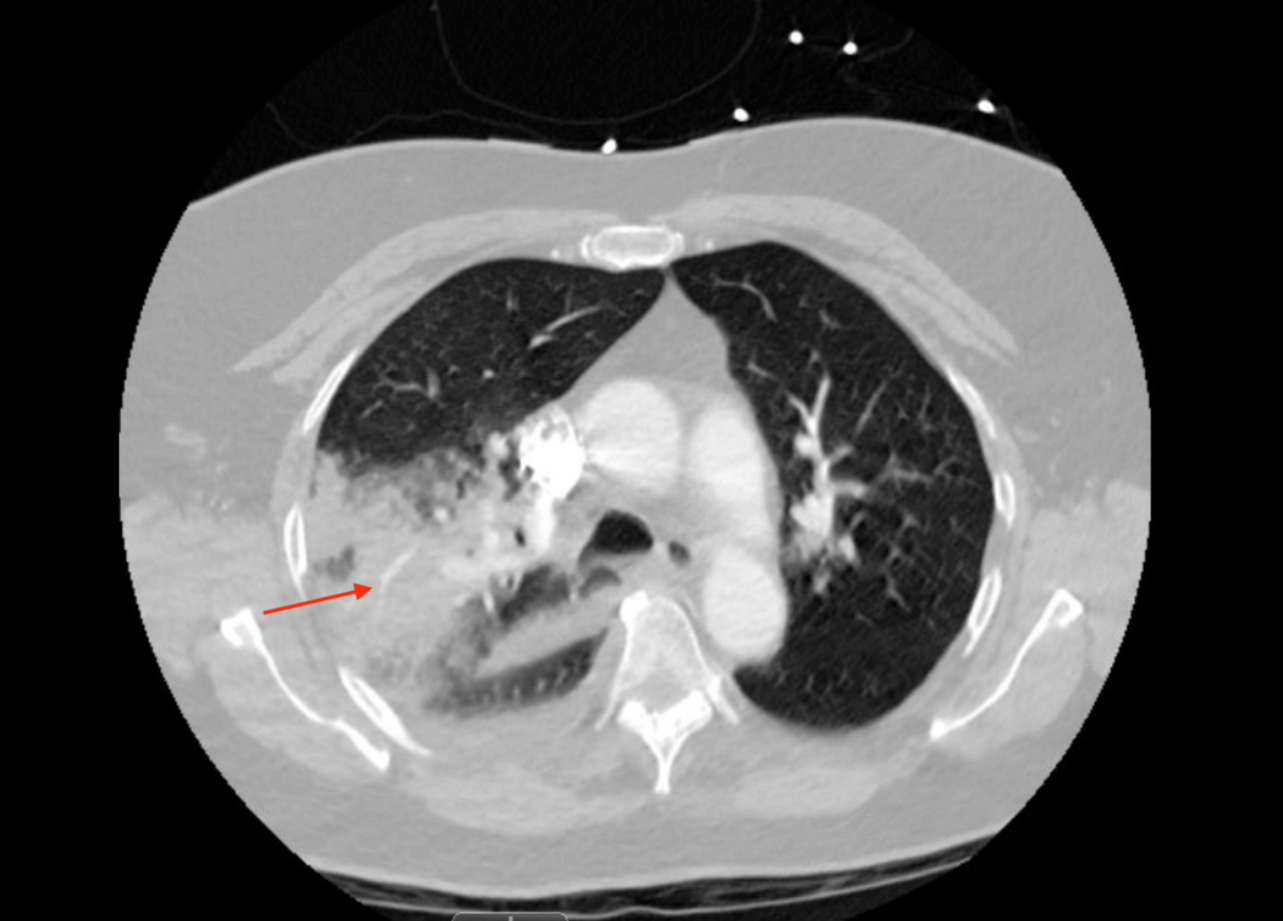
Repeat CT Angiography Post-thrombectomy Interval evacuation of right main and lobar pulmonary arterial emboli. Extensive consolidation within the right upper lobe with restored contrast opacification demonstrating patent blood flow.
